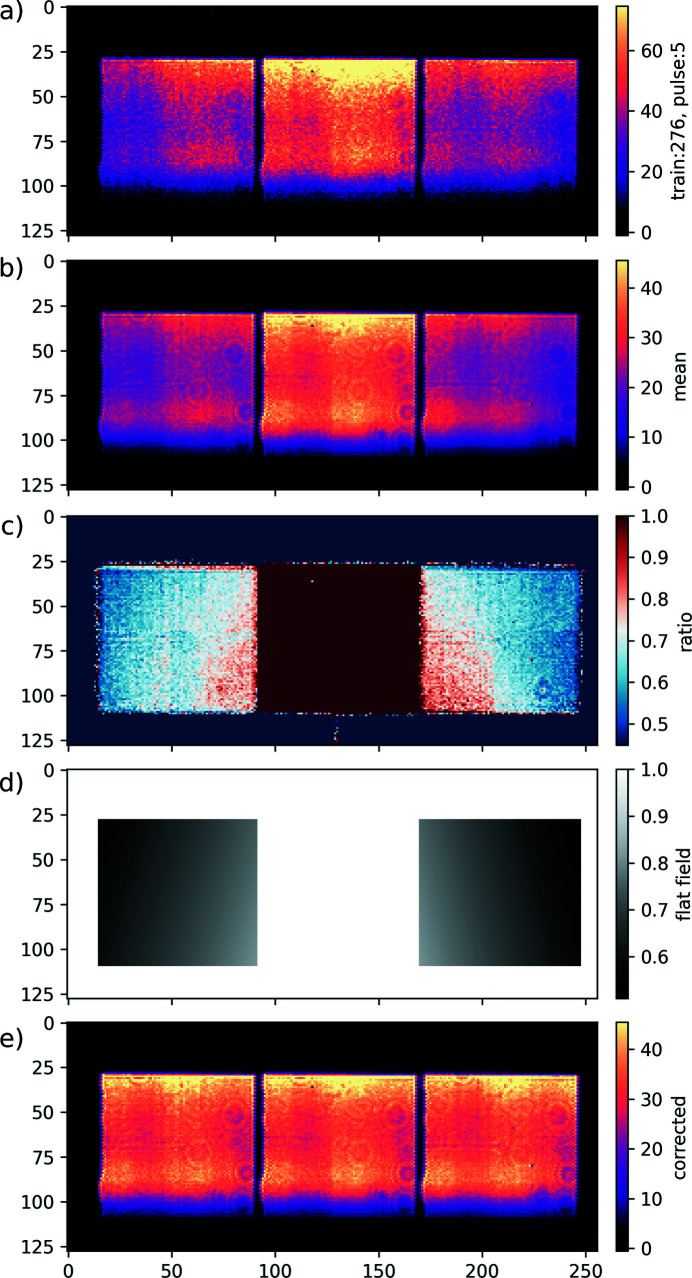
(*a*) Dark-corrected single-pulse image of the DSSC sensor showing the three imaged beams (−1st, 0th and +1st order). (*b*) Dark-corrected average image of a single DSSC sensor showing the three imaged beams based on a 5 min-long data acquisition recording 3000 FEL trains with 15 X-ray pulses per train. (*c*) Image showing each beam normalized by the 0th order beam. (*d*) Fitted flat-field correction. (*e*) Dark-corrected and flat-field-corrected average image in a single DSSC sensor. The three imaged beams now appear as identical copies of the initial beam.
